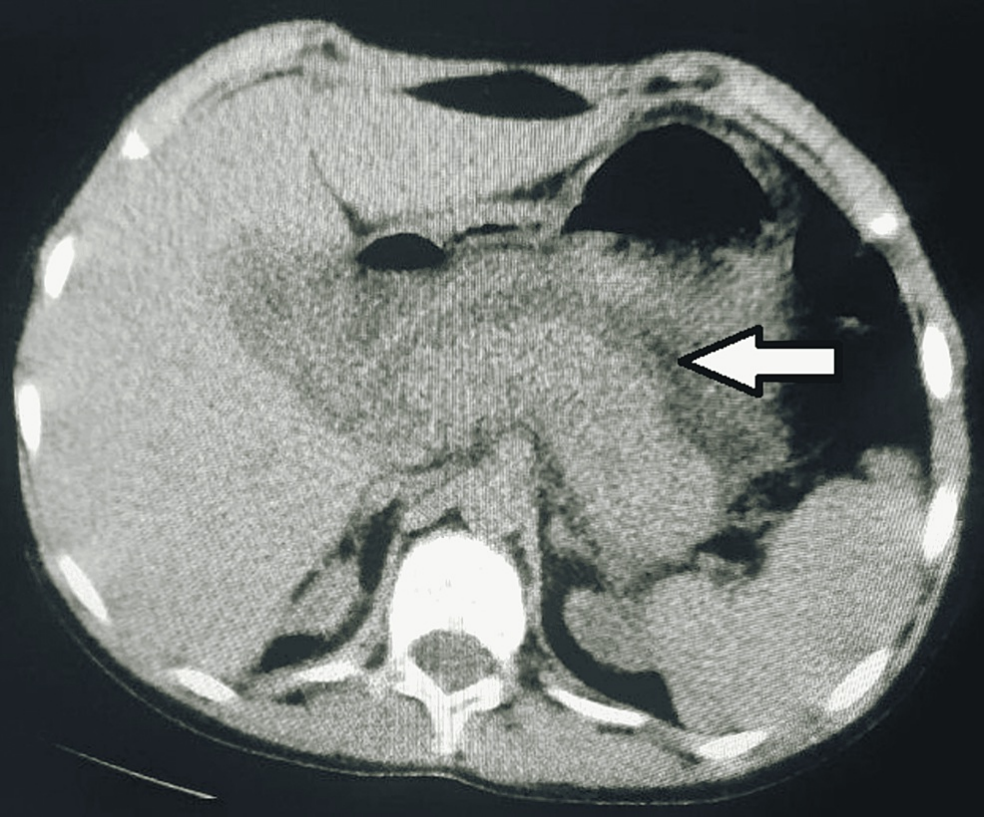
Abdominal CT demonstrating diffuse pancreatic enlargement with peripancreatic fluid and fat stranding (white arrow).
